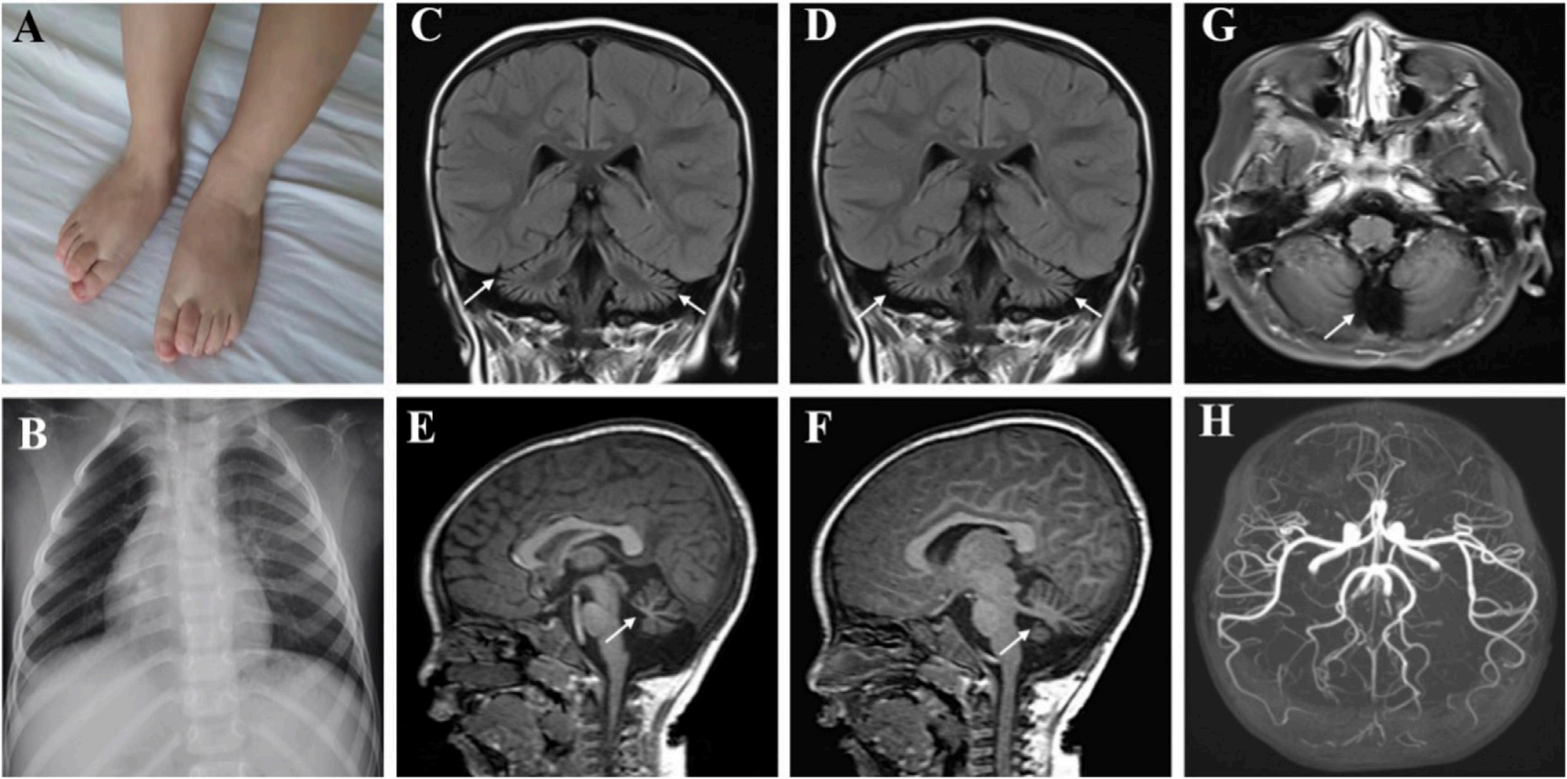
Clinical features of the patient with *MSTO1* variant. The second toes of both feet were upward deformity (A). Chest radiograph showed increased brightness of the right lung, hyperventilation was considered (B). (C–F) Brain MRI revealed reduced cerebellar volume, deepened sulci and fissures, and narrowed gyri, the fourth ventricle and occipital cisterna were enlarged (G). MRA was normal (H).
